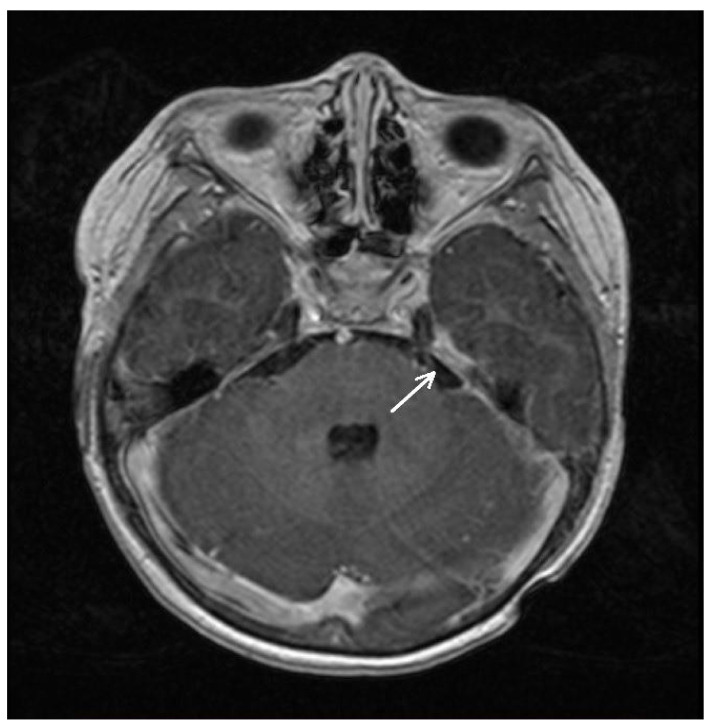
Axial MRI of the brain (T2 weighted). The arrow indicates the site of leptomeningeal metastasis of the tumor.
